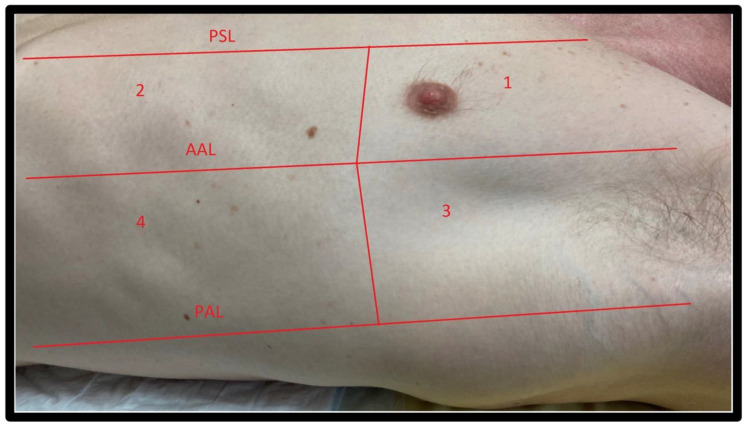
International Consensus Conference for point-of-care lung ultrasound areas. Four chest areas per side considered for complete eight-zone lung ultrasound examination (modified from Volpicelli et al. [5]). AAL: Anterior Axillary Line; PAL: Posterior Axillary Line; PSL: Parasternal line.
